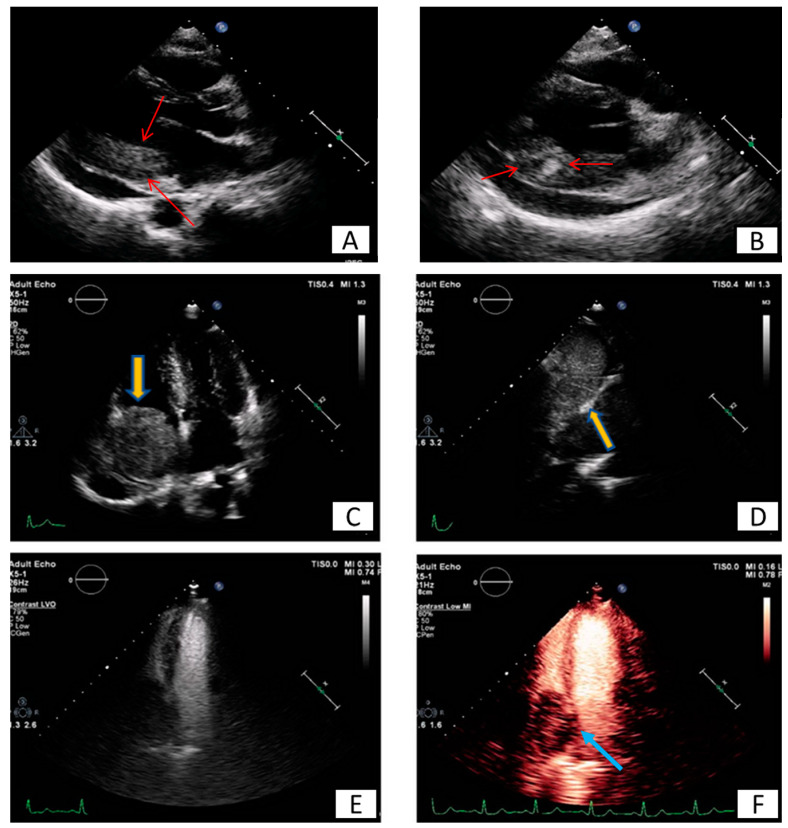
Long (A) and modified short axis (B) view of transthoracic echocardiography in a young female patient with metastatic carcinoma. Note the presence of pericardial effusion and the increased echogenicity and inhomogeneity of the basal posterior myocardial wall finding consistent with malignancy (red arrows). (C–F) Patient presenting with dyspnea on exertion and right heart failure symptoms during last months. A large mass (5.2 × 4.8 cm) is depicted inside the right atrium (C), originating from inferior vena cava (D). Utilizing echo contrast agents, the increased vasculature of the mass is revealed, a finding supportive of malignancy (E,F).
